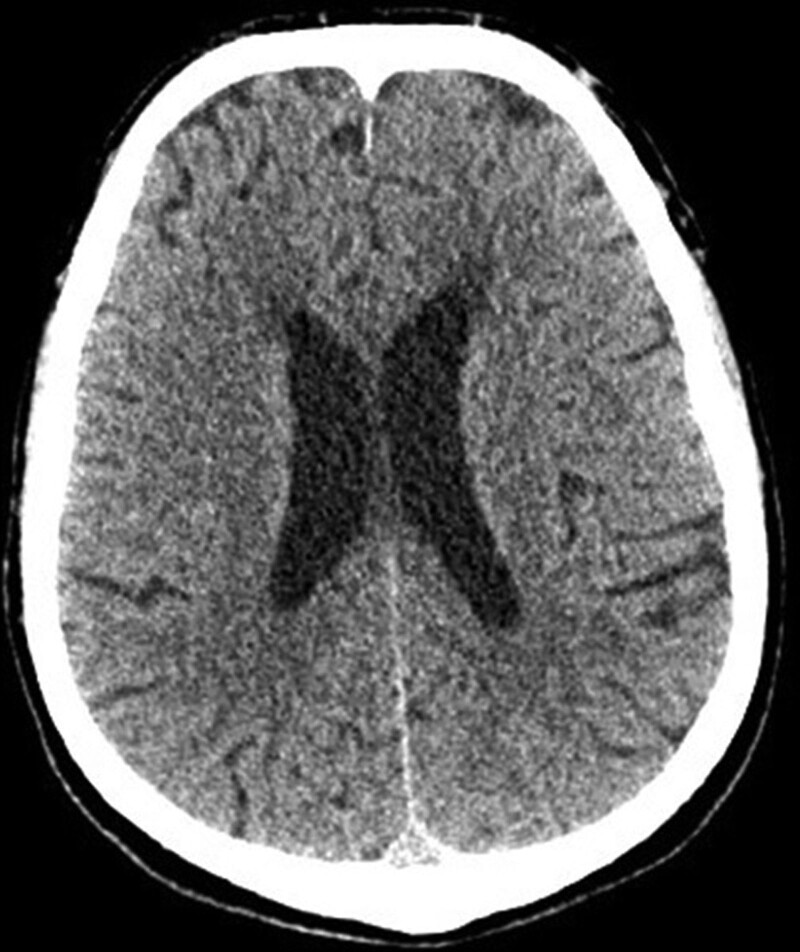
Head CT scan of the patient at first hospitalization.
